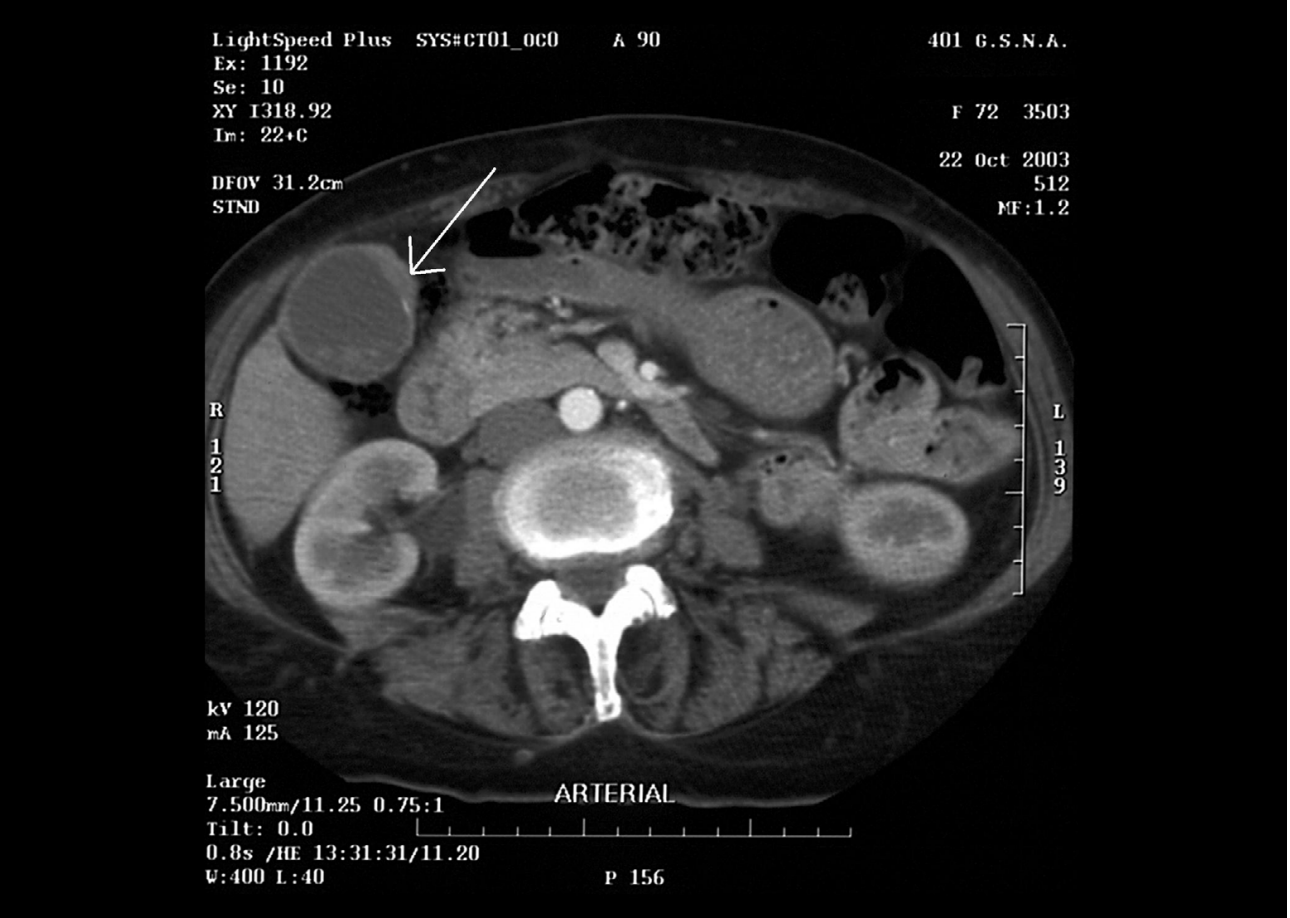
Computed tomography, showing cholelithiasis with thickening of gallbladder anterior wall (arrow in the figure).
